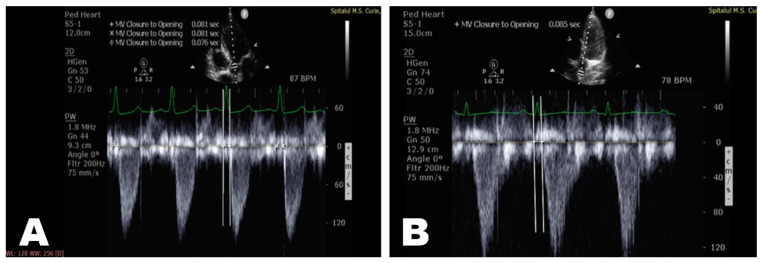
Measurement of ΔT1 (T1-T0 = 85 − 81 = 4 msec) in the ascending aorta (echocardiography—apical window 5C) (A,B).
